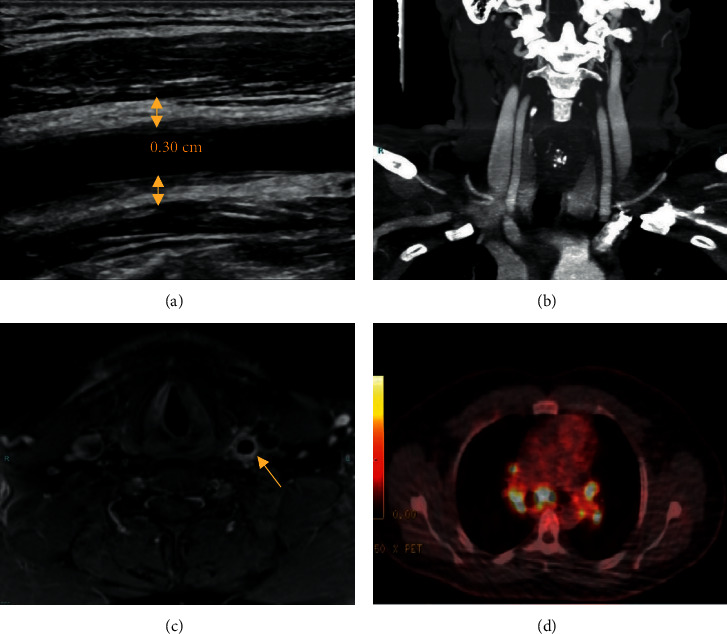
(a) Longitudinal ultrasound view showing LCC wall thickening (arrows). 0.30 cm relates to vessel wall thickness. (b) Coronal CTA view showing no LCC wall thickening. (c) Axial MRI view showing prominent LCC wall thickening. (d) PET/CT scan showing hypermetabolic mediastinal and hilar lymphadenopathy. ^∗^Dimensions not to scale. CTA : computed tomography angiography; LCC : left common carotid; PET : positron emission tomography; MRI : magnetic resonance imaging.
